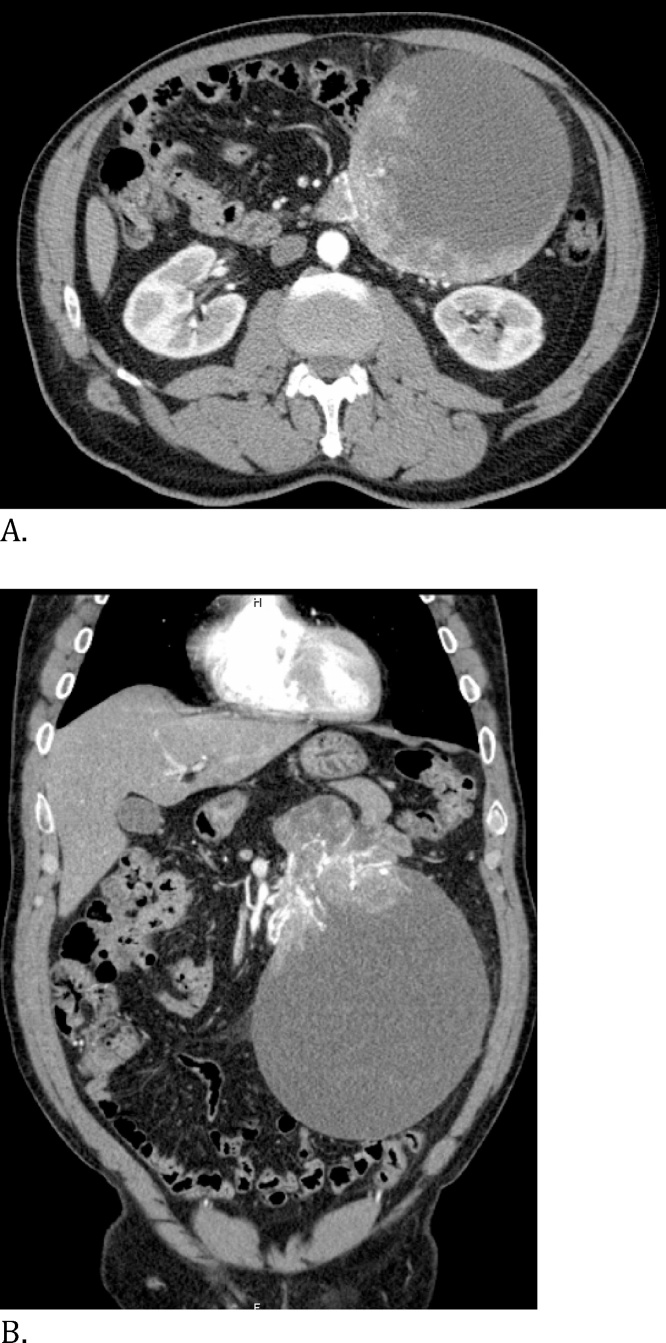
CT scan showing a large tumor arrising from the first jejunal segment: A. Sagital image; B. Coronal image.
